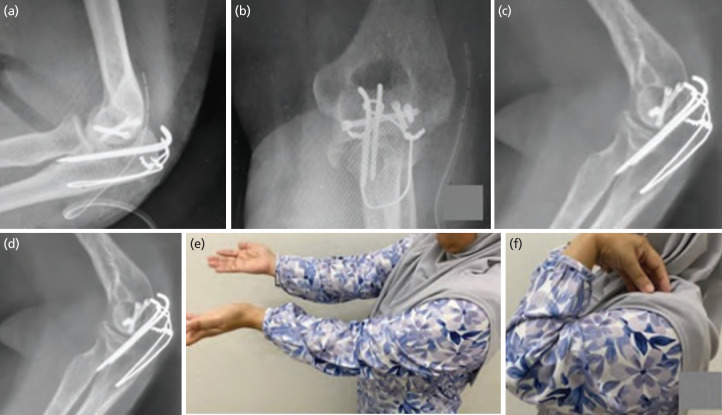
(a, b) Immediate post operative radiograph shows acceptable fracture fragment reduction with congruent articular surface. (c, d) United olecranon and coronal shear fracture with congruent articular surface at six months after fixation. (e, f) Post-operative range of motion.
